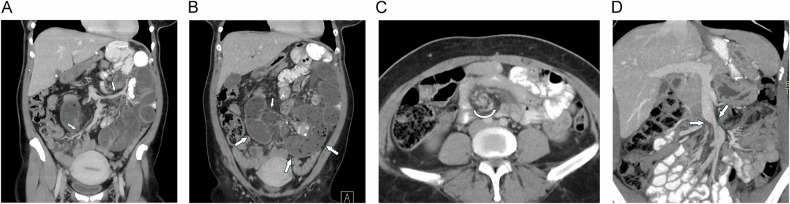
CT signs of internal hernia. A Coronal MRP shows deviated SMV with dilated tributaries (small arrows). B The small bowel loops in the mid-abdomen are dilated and C-shaped (large arrows), and there is mesenteric edema (small arrow). C In another patient, axial MDCT shows typical rotation of the mesenteric vessels around their axis (curved arrow) (“whirl sign”). D In the same patient, coronal MIP shows beak-like constriction of SMV due to torsion (arrows)
